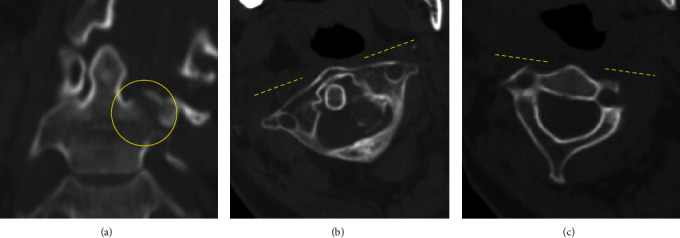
CT after the second reduction, revealing the fracture of the C2 lateral mass (yellow circle). (a) Axial view of C1, (b) axial view of C2, and (c) coronal view. Atlantoaxial rotatory subluxation remained, although the C1-C2 rotatory angle decreased to 30 degrees.
